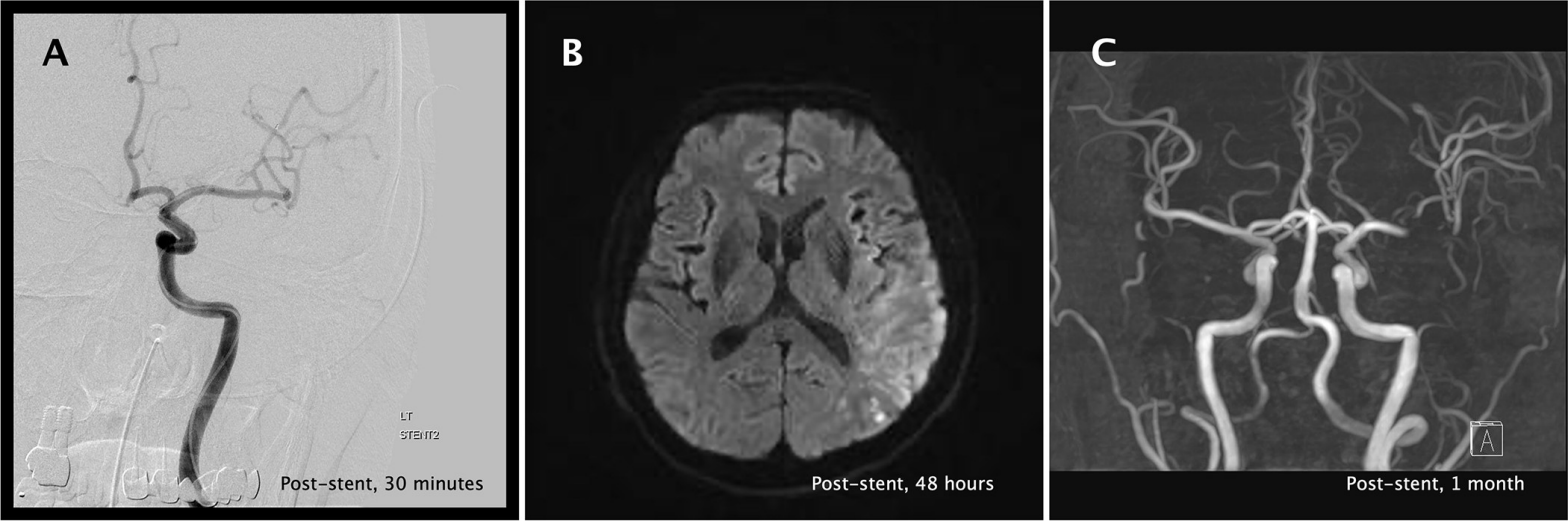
Radiologic images of a patient before and after RS deployment. Before IAT, diffusion-perfusion mismatch was identified by comparing the DWI to CTP images (A). Three months after the procedure, recanalization of M2 was noted on CTA. RS, rescue stent; IAT, intra-arterial thrombectomy; DWI, diffusion-weighted image; CTP, perfusion computed tomography; CTA, computed tomographic angiography.
